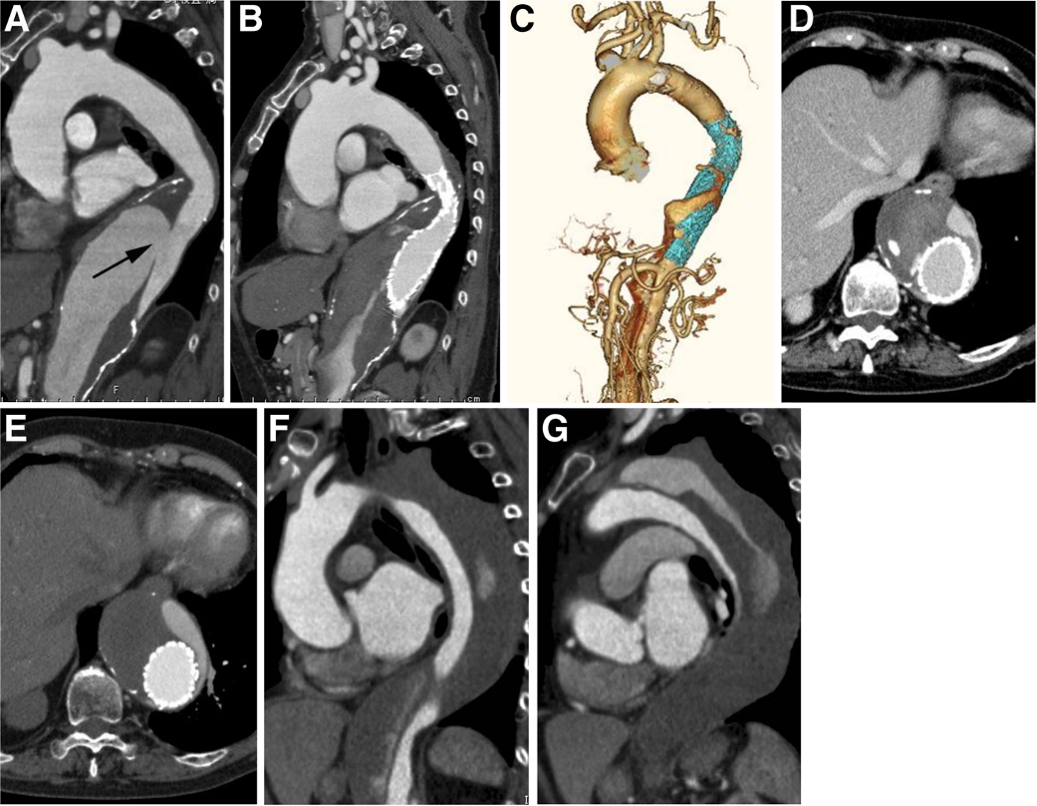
A 78-year-old female with type B chronic AD. A. Six years after onset, contrast-enhanced CT image shows aneurysmal dilation of the affected aorta and entry site (arrow). B, C. TEVAR (GORE TAG: 37-mm diameter & 20-cm length) was performed. Contrast-enhanced CT image and 3D CT angiography show that the entry site was closed. D, E. Six (F) and (G) 15 months after TEVAR, contrast-enhanced CT images show that the false lumen has partially thrombosed, but aneurysmal dilation of the affected aorta remains.
